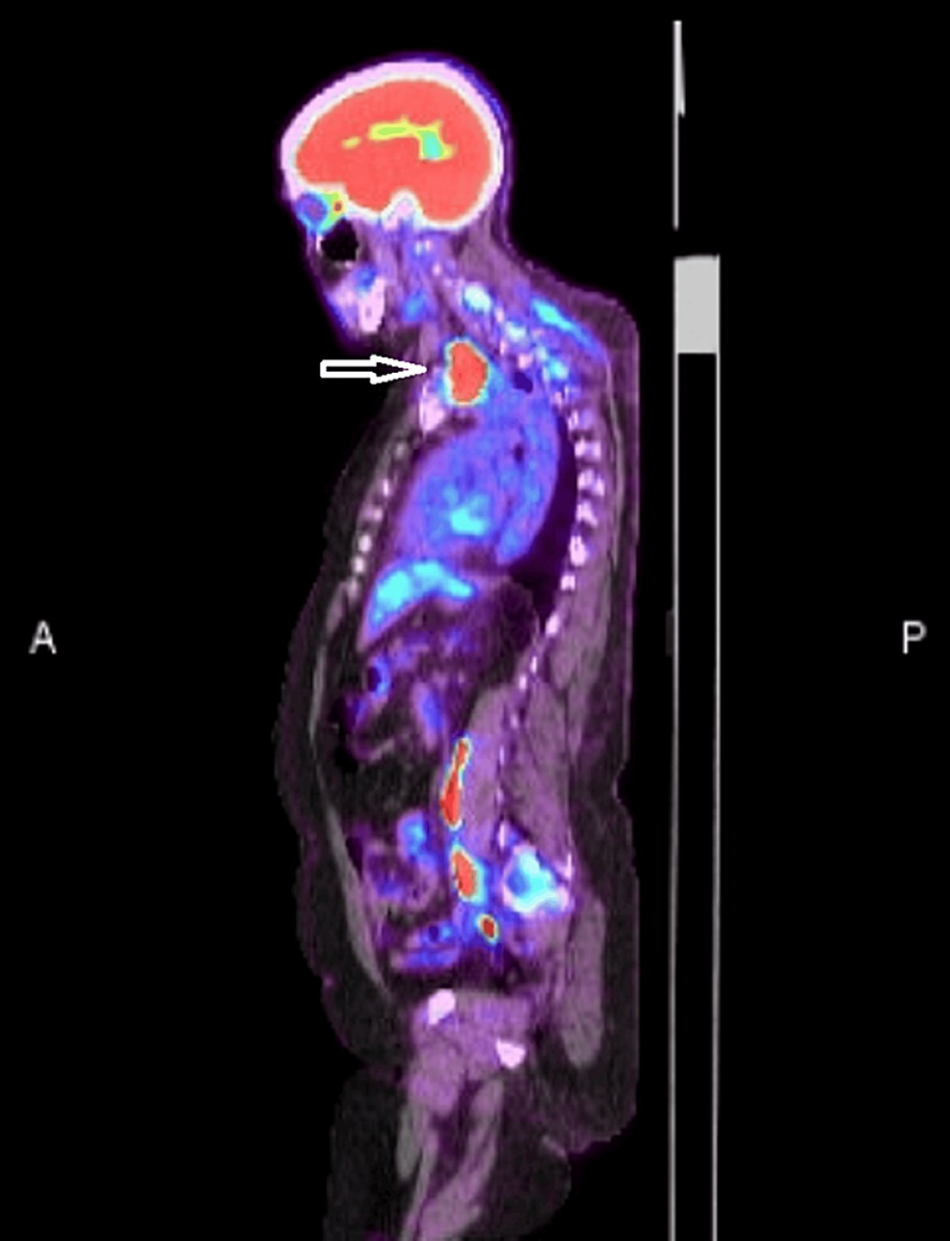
Upper mediastinal mass with high FDG uptake. FDG: fluorodeoxyglucose
